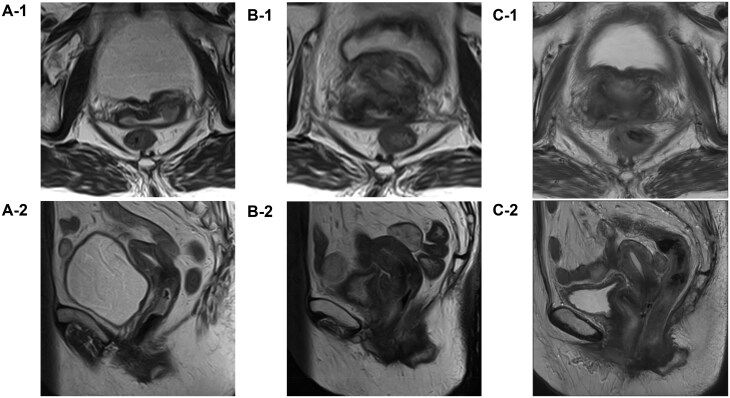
T2-weighted magnetic resonance imaging (A) before treatment, (B) after 3 courses of the QUAD shot, and (C) 6 months after completion of 2 courses of brachytherapy. (A-1, B-1, C-1) Axial and (A-2, B-2, C-2) sagittal plane images are shown. (A-1) Rupture of the cervical stromal ring in the left parametrium. (A-2) Vaginal extension of the cervical mass. (B) The vaginal extension is no longer evident, but the cervical mass appears to be partially residual. (C) The cervical mass is no longer visible.
